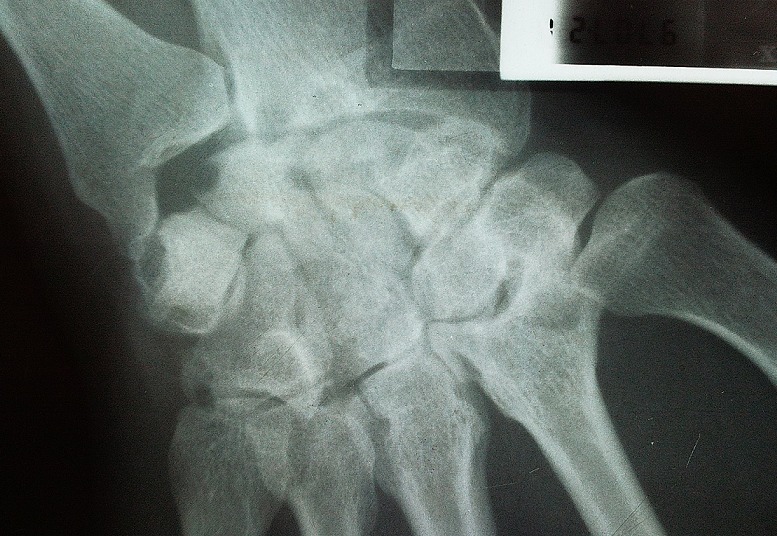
Radiographie de la main droite: carpite droite stade 3 (érosions avec pincement des os du carpe)
